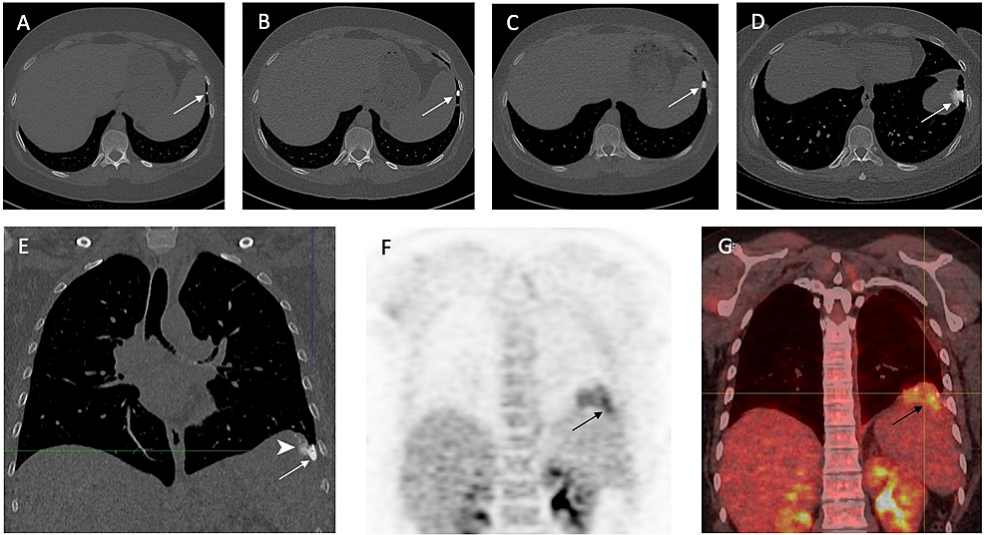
Lung metastasis Initial chest CT scan (A) in 2016 revealed a small calcified lesion in the left lower lobe (white arrow). The lesion remained relatively stable in appearance and size on follow-up chest CT scans in 2018 (B) and 2019 (C), suggestive of a benign process. Axial (D) and coronal (E) chest CT scan in 2021 shows an increase in the size of the lesion with diaphragm invasion (white arrowhead). Coronal 18F-fluorodeoxyglucose positron emission tomography (F) and fused scan (G) images show increased activity of the lesion (black arrow).
